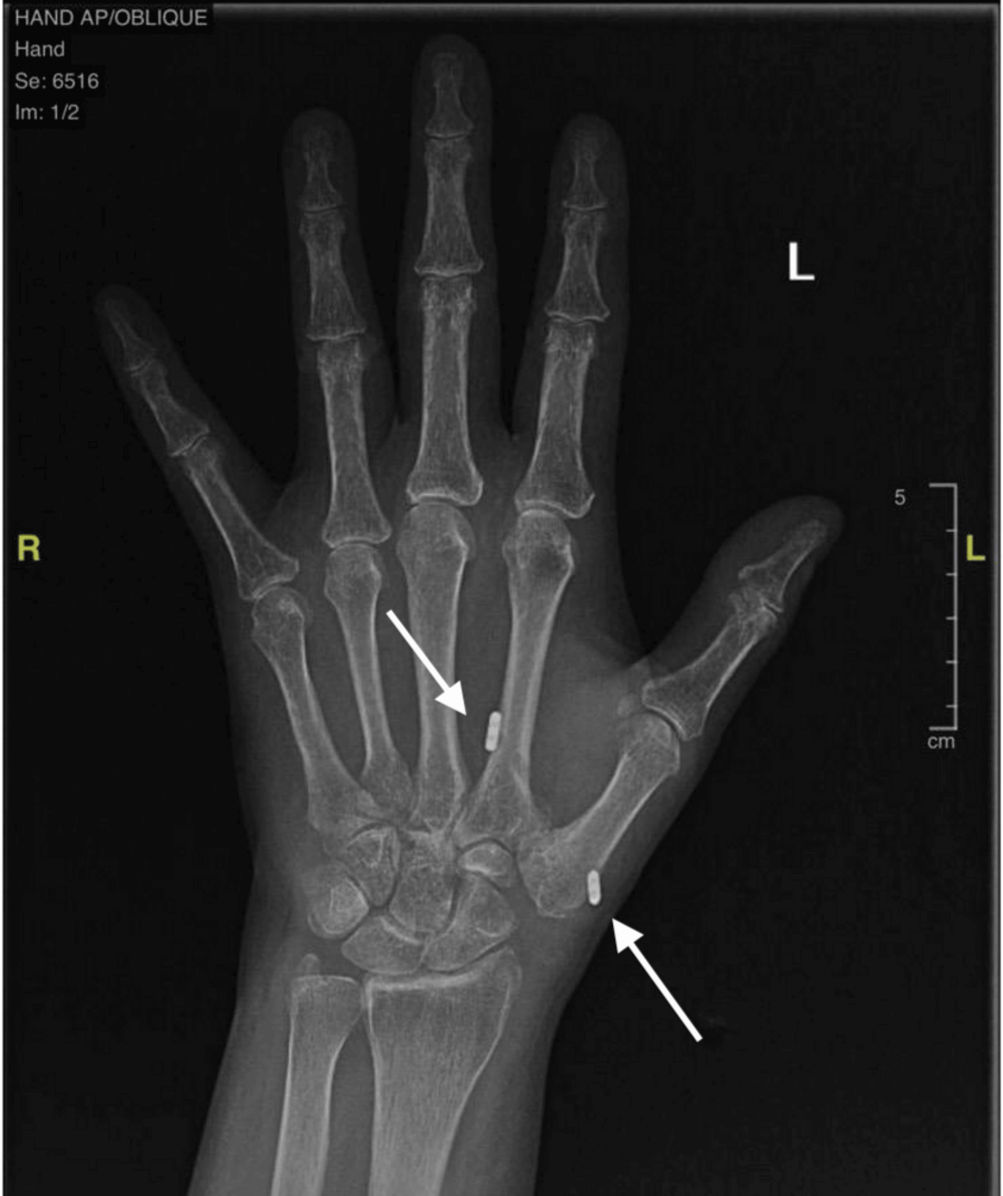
Anteroposterior (AP) X-ray of the hand following trapeziectomy with Mini TightRope fixation, with arrows indicating the Mini TightRope fixation points.
